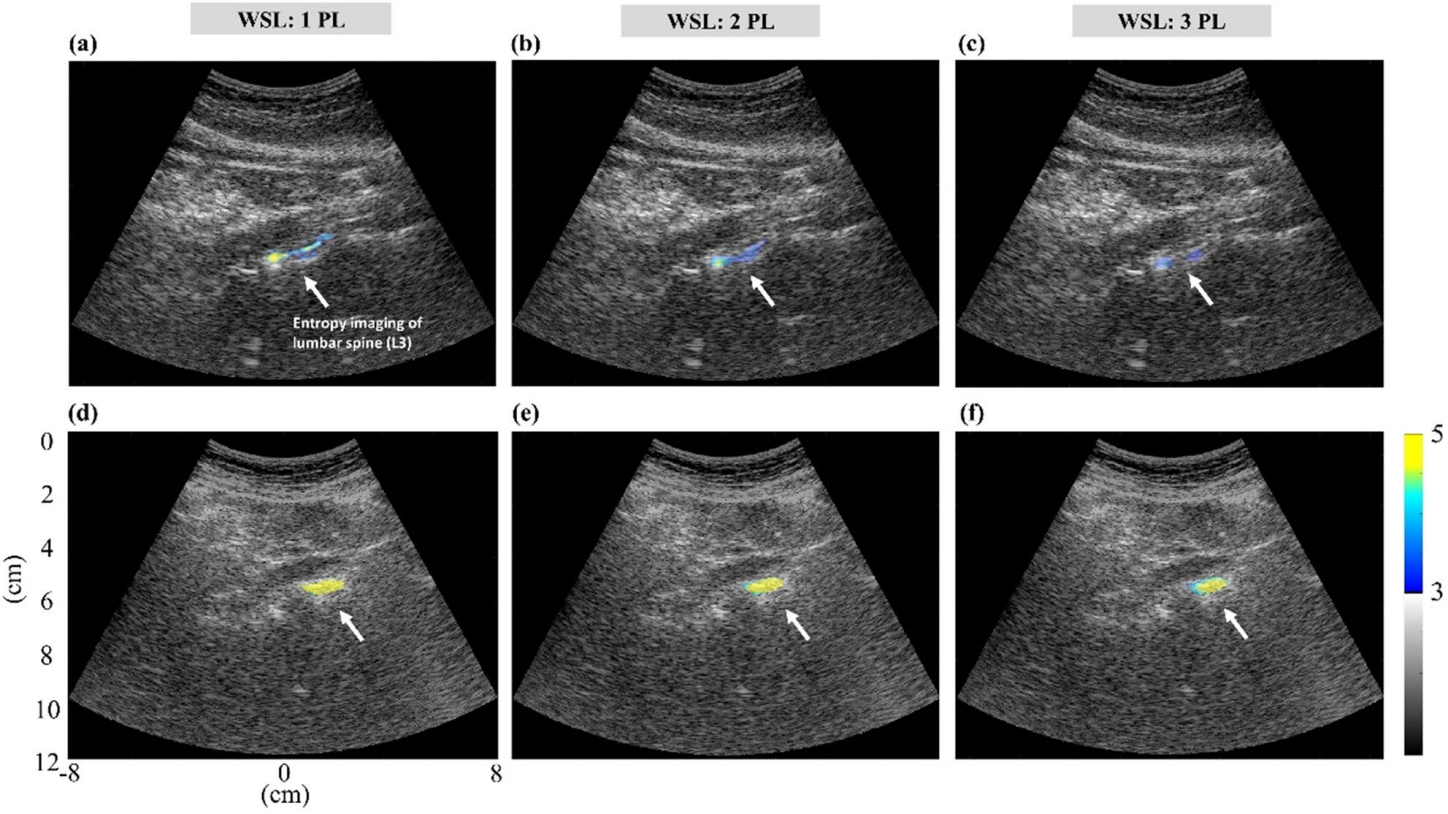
Typical ultrasound entropy images constructed using different WSLs superimposed onto the corresponding B-mode images for (a–c) Group I participant and (d–f) Group II participant.
